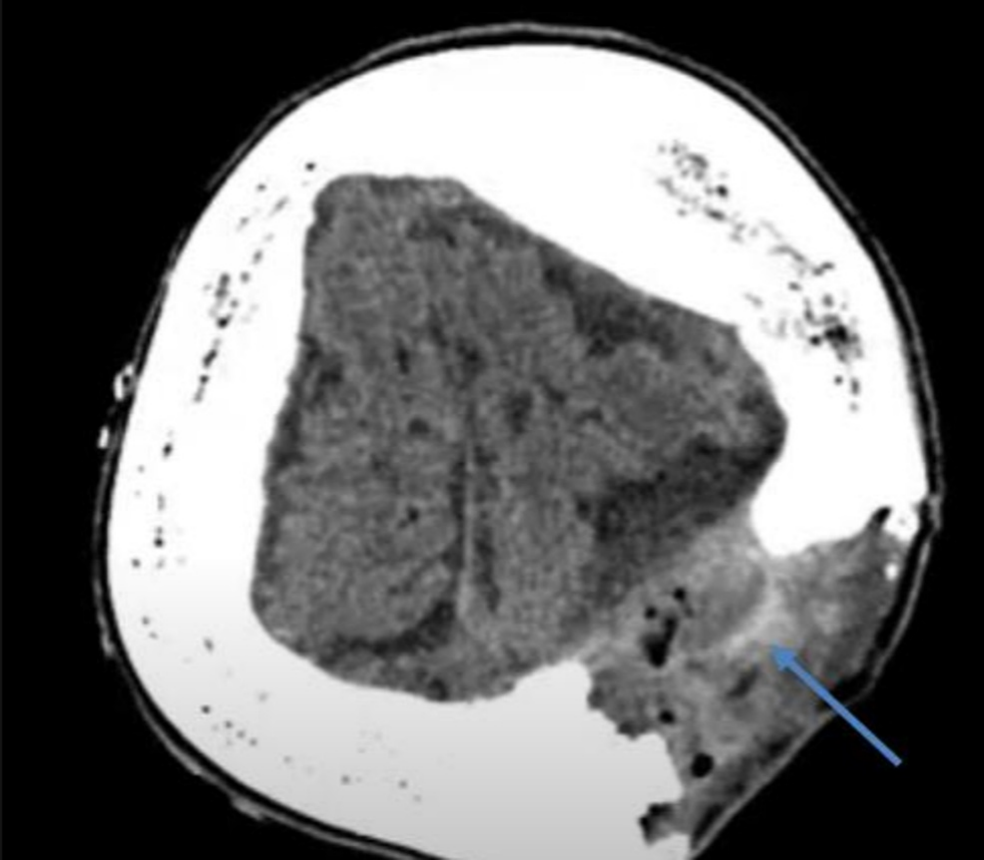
CT brain done showed redemonstration of operative bed subgaleal and epidural heterogenous marginally enhancing collection measuring 7 x 4.5 x 4.5 cm (pointed in the image).
